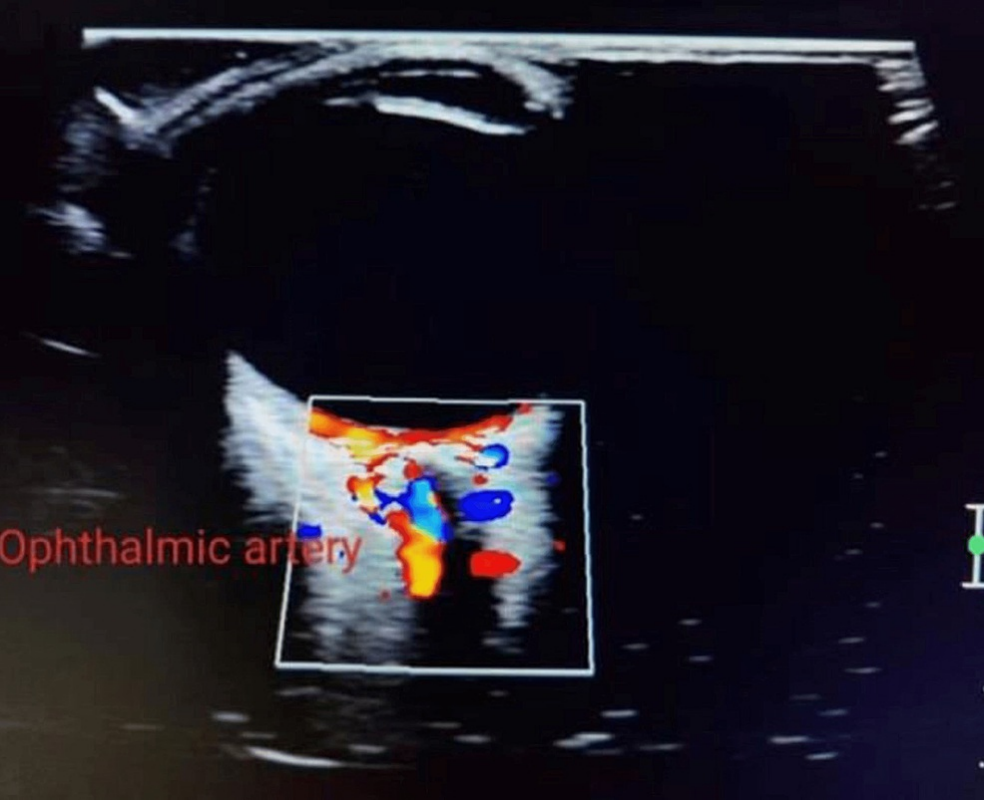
Ophthalmic artery in colour Doppler
